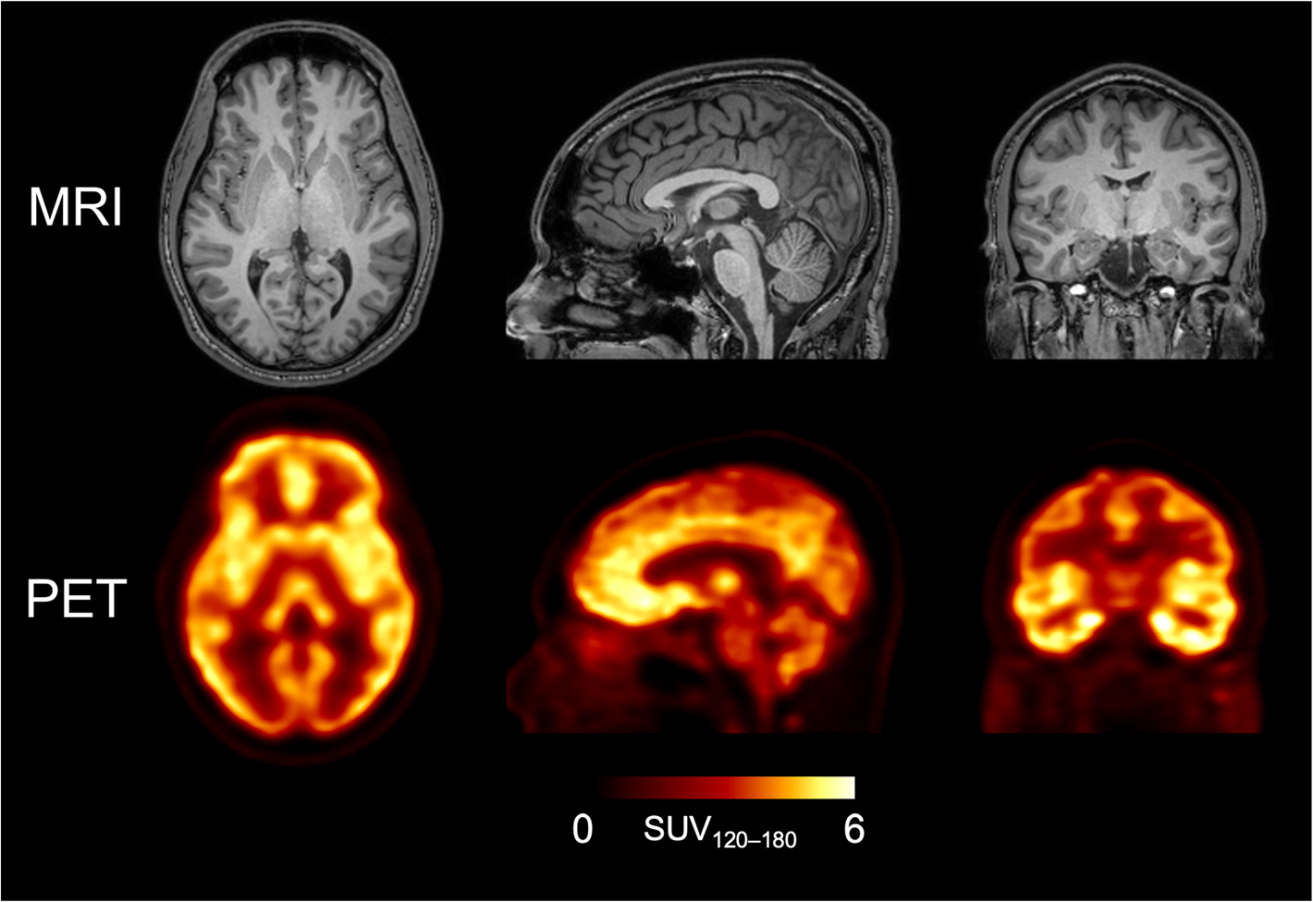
T1-weighted magnetic resonance (MR) and [^18^F]LSN3316612 positron emission tomography (PET) images of a healthy volunteer. Although the brain had high uptake of radioactivity, the skull had virtually none. The concentration of radioactivity in the brain is expressed as standardized uptake values (SUV), which was measured from 120 to 180 min
